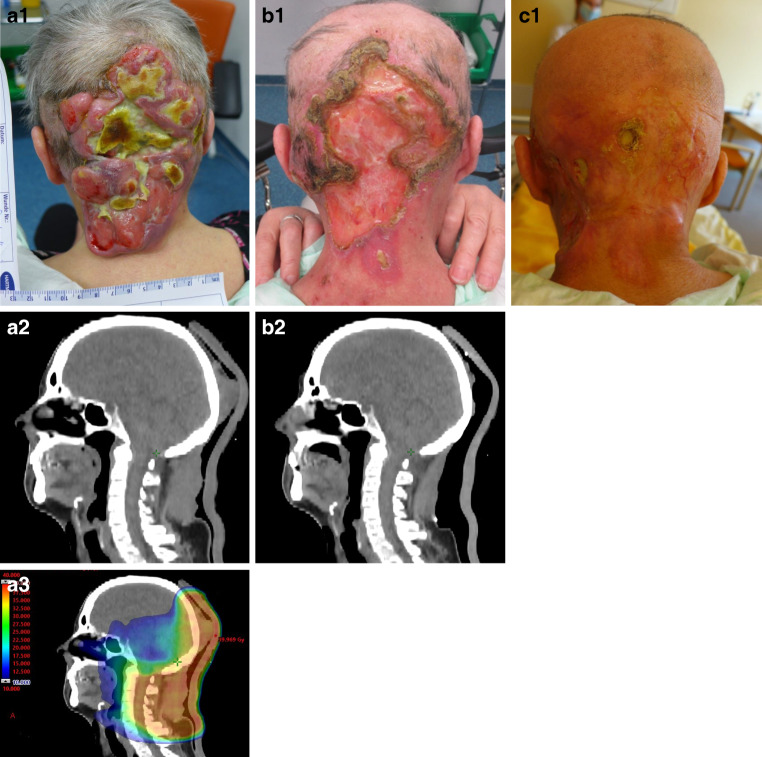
a–c Example of patient with malignant wound responding well to RT (tumor mass reduction and improvement in wound conditions) and achieving the treatment goal: (Follicular B‑cell lymphoma, initial stage IIIB, palliative RT). (*a1*) Wound documentation before RT, (*a2*) axial slice of the pre-treatment RT planning CT, (*a3*) VMAT-radiotherapy plan, dose color wash ranging from 10 Gy (blue, lowest value) to 39,969 Gy (maximum dose, red) on this plane. (*b1–2*) Wound documentation and CT-scan at the end of RT and (*c*) wound documentation 3.5 months after RT
